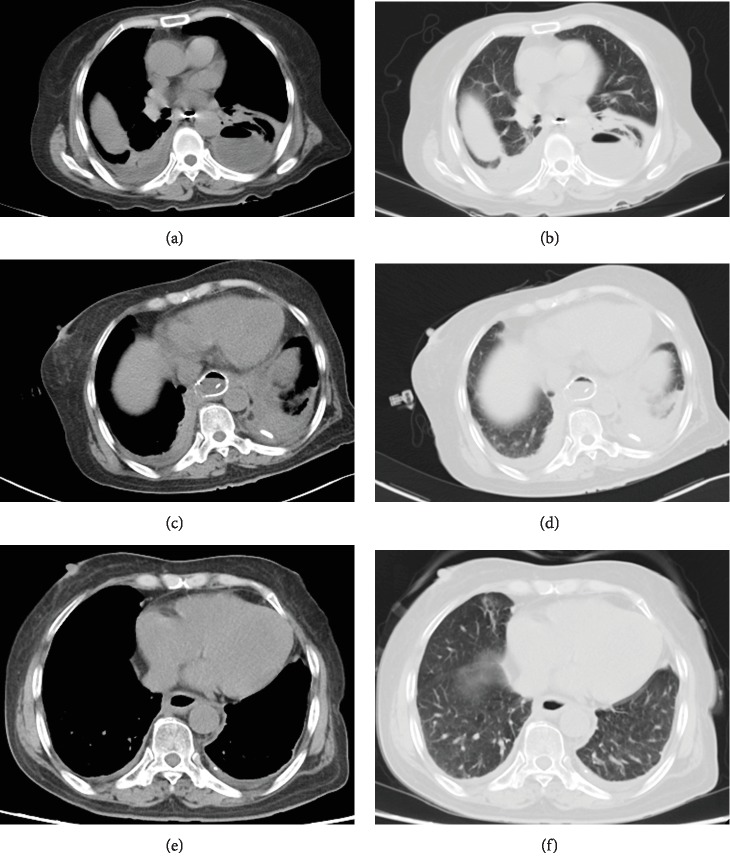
Chest computed tomography for case 16. (a, b) Chest CT scan in the mediastinal and lung windows shows anastomotic leakage, atelectasis, and bilateral pleural effusion before interventional procedure. (c, d) At one month after three-tube treatment and covered stent placement, a chest CT scan shows a covered stent with decreased pleural effusion. (e, f) After removal of three-tube and covered stent, a chest CT scan shows disappearance of abscess, expansion of the lungs with no pleural effusion.
